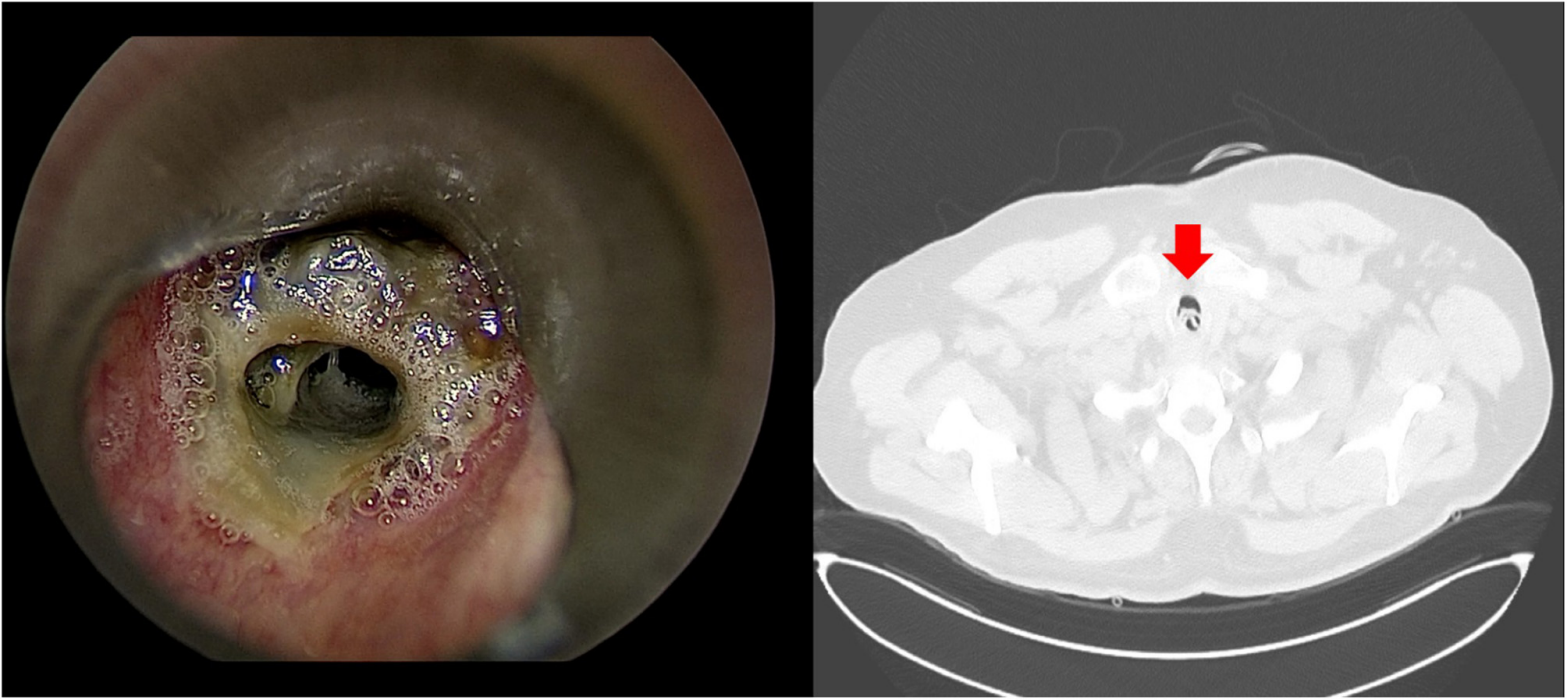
Bronchoscopic view and the axial CT scan of patient with obstructive fibrinous tracheal pseudomembrane.
